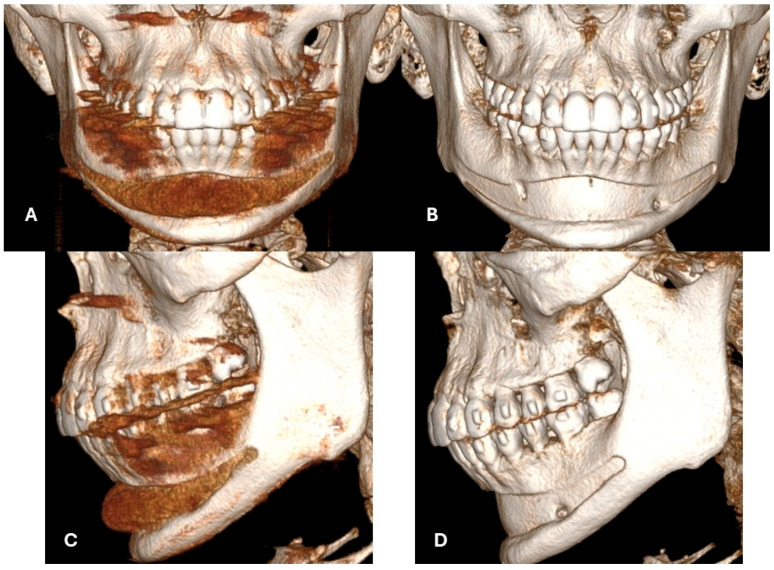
A 21-year-old Caucasian woman who presented for consultation after a failed stock chin-implant placement elsewhere. Clinically, she exhibited Class II malocclusion with vertical overgrowth of the anterior maxilla resulting in a gummy smile, recessed chin, overbite, and retrognathia. She was undergoing orthodontic treatment with removable (camouflage) appliances at the time of consultation. Orthodontic camouflage and implant placement had been attempted to mask the major craniofacial and dental arch relationship anomalies. CT imaging showed the chin implant was positioned inappropriately high relative to the mental protuberance and not on the inferior mandibular border (A–C). This led to implant collapse into the mandibular groove and inadequate soft-tissue projection (B). The implant had been placed passively without stabilization, which caused significant bone resorption along the implant, extending to the apices of the anterior teeth (B,D).
